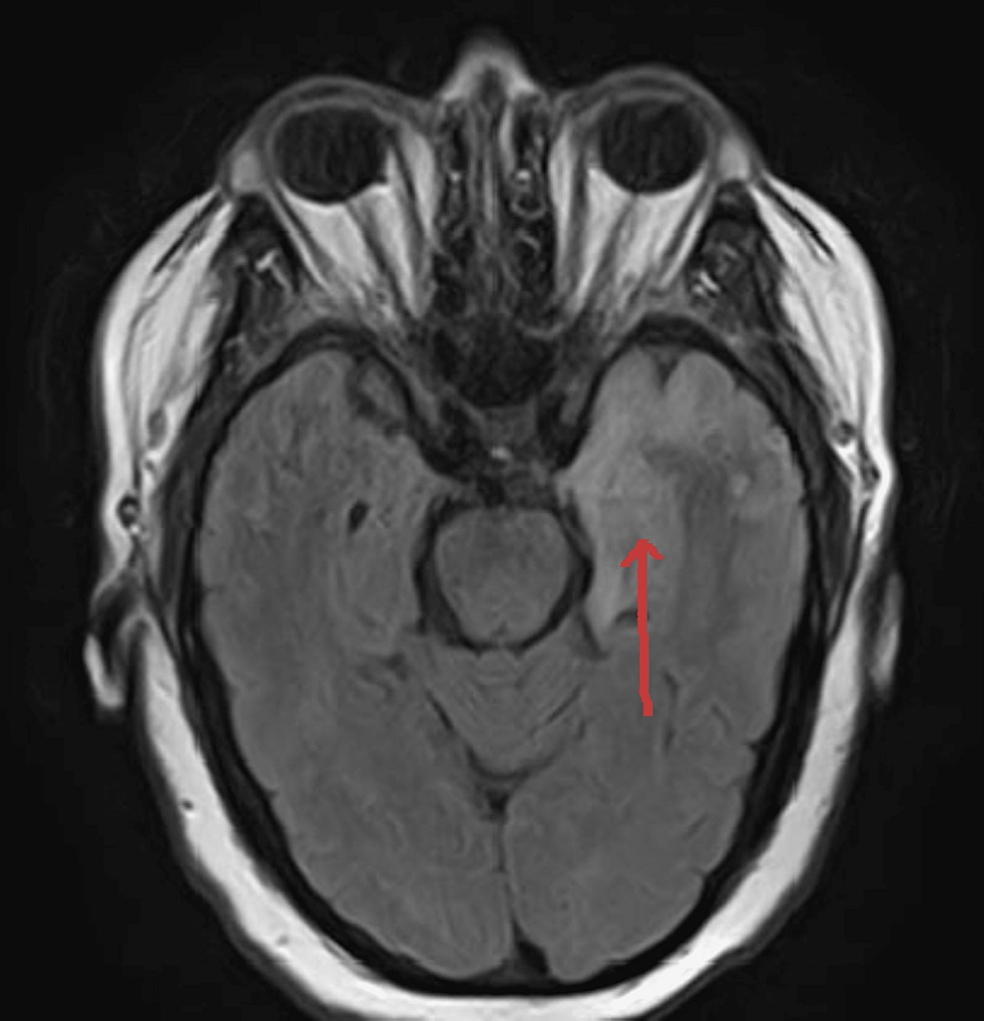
MRI scan, FLAIR phase An abnormal high signal was seen on FLAIR involving the left insular cortex and left temporal lobe. FLAIR: fluid-attenuated inversion recovery.
